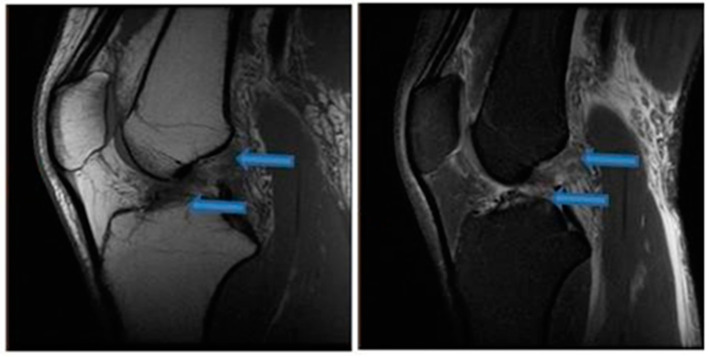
Sagittal T1- and T2-weighted magnetic resonance imaging of the knee demonstrating ACL detachment at the femoral origin indicated by blue arrows (20 July 2020).
